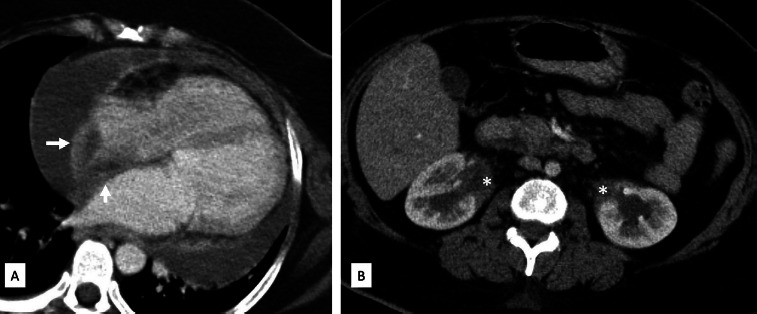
Delayed CT image in 4 chamber view (A) shows right atrial and right atrioventricular groove mass lesions which are similar to those seen on cardiac MRI. Abdominal CT axial image (B) shows bilateral perirenal soft tissue in parapelvic location (the white asterisks) encasing the renal hilar structures with resultant bilateral hydronephrosis
